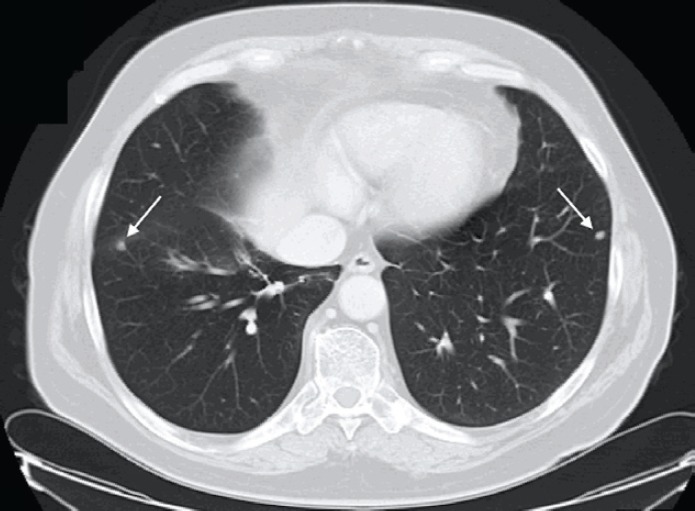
Patient #1. Thoracic CT scan documenting micronodules in the right and left lungs (lower lobes). Arrows indicate secondary lesions.
